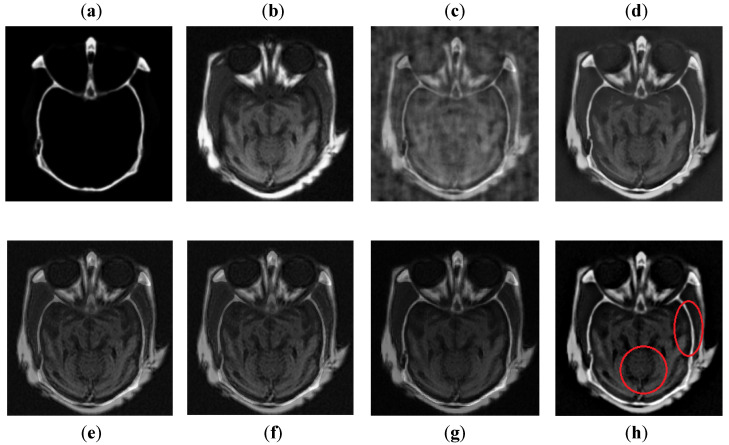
Data-1: (a) CT image, (b) MRI image, (c) DCT, (d) DWT, (e) NSST-SFT-PCNN, (f) CSR, (g) CNN, and (h) Proposed.
